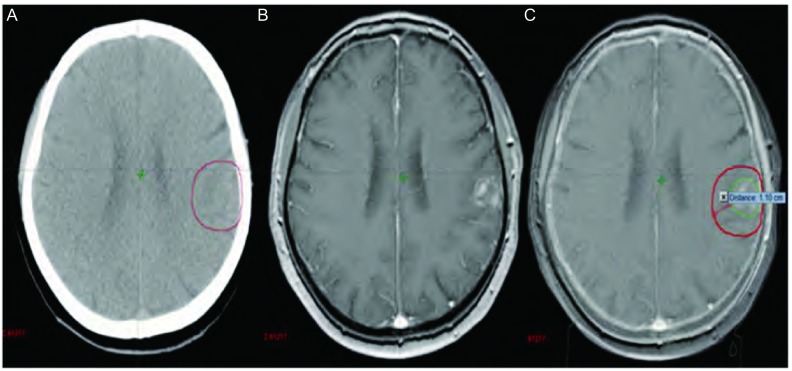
幕上转移灶（A：增强CT图像；B：MRI图像；C：CT/MRI融合图像）。 Supratentorial metastasis. (A: Enhanced CT image; B: MRI image; C: CT/MRI fusion image).
